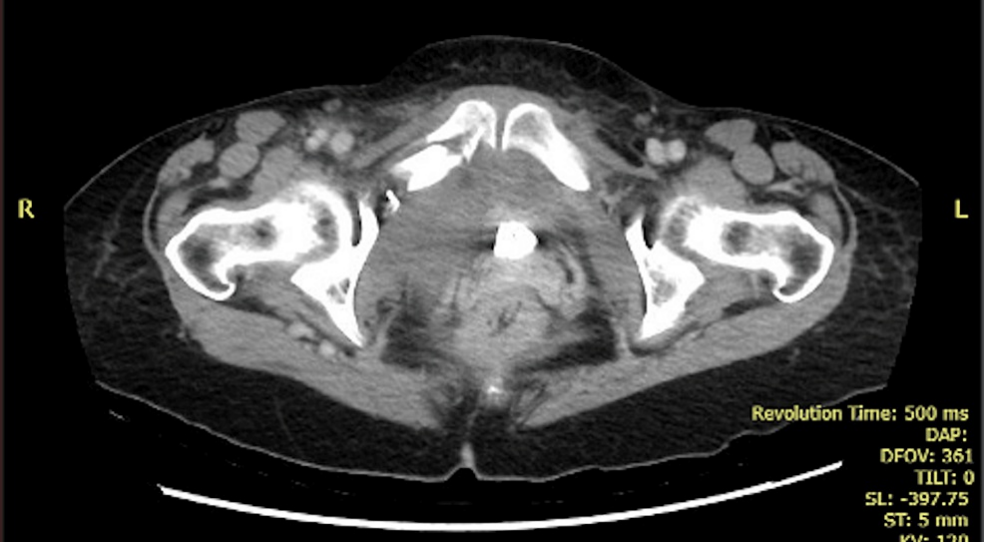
A CT of the abdomen and pelvis with contrast exhibiting an absence of delayed contrast extravasation in the pelvic hematoma CT: computed tomography
